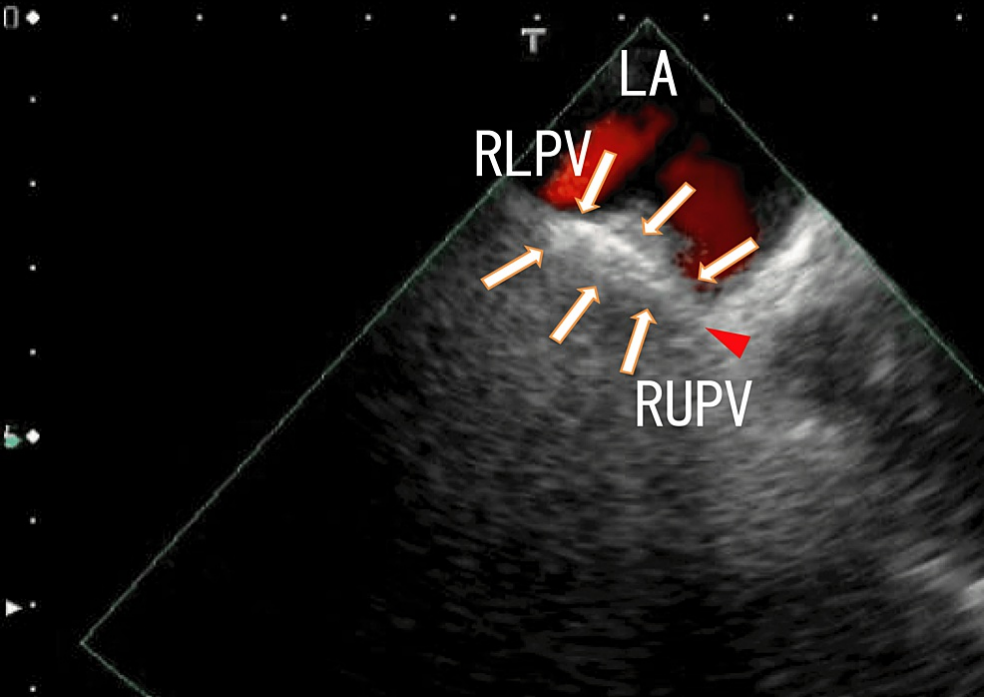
TEE images demonstrating thrombi in the RLPV attached to the LA wall TEE images demonstrate thrombi in the RLPV as white thick line-like shapes that appeared to extend to the LA (arrows) and attached to the anterosuperior wall of the LA (arrowhead). The thrombi included a thick white area and were linear in shape. The blood flow from the RLPV is shown as a bright red area, and the blood flow from the RUPV is shown as a dark red area. TEE, transesophageal echocardiography; LA, left atrium; RLPV, right lower pulmonary vein; RUPV, right upper pulmonary vein.
